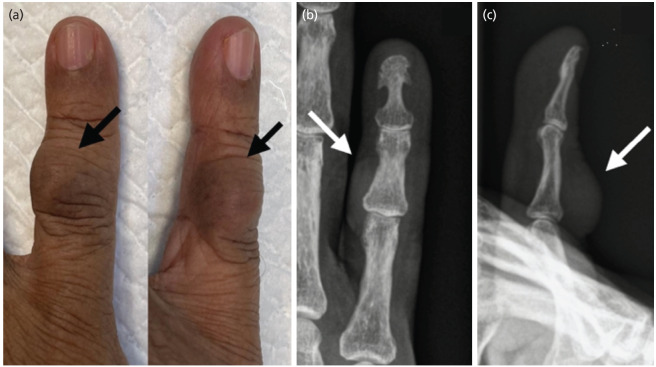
(a) Clinical photograph showing the dorsal aspect of the right little finger with swelling (black arrow) over the PIPJ with no skin changes and smooth surface. (b) Plain radiograph of the right little finger in anterior-posterior view and (c) lateral view showing no bone involvement and soft tissue swelling (white arrow).
